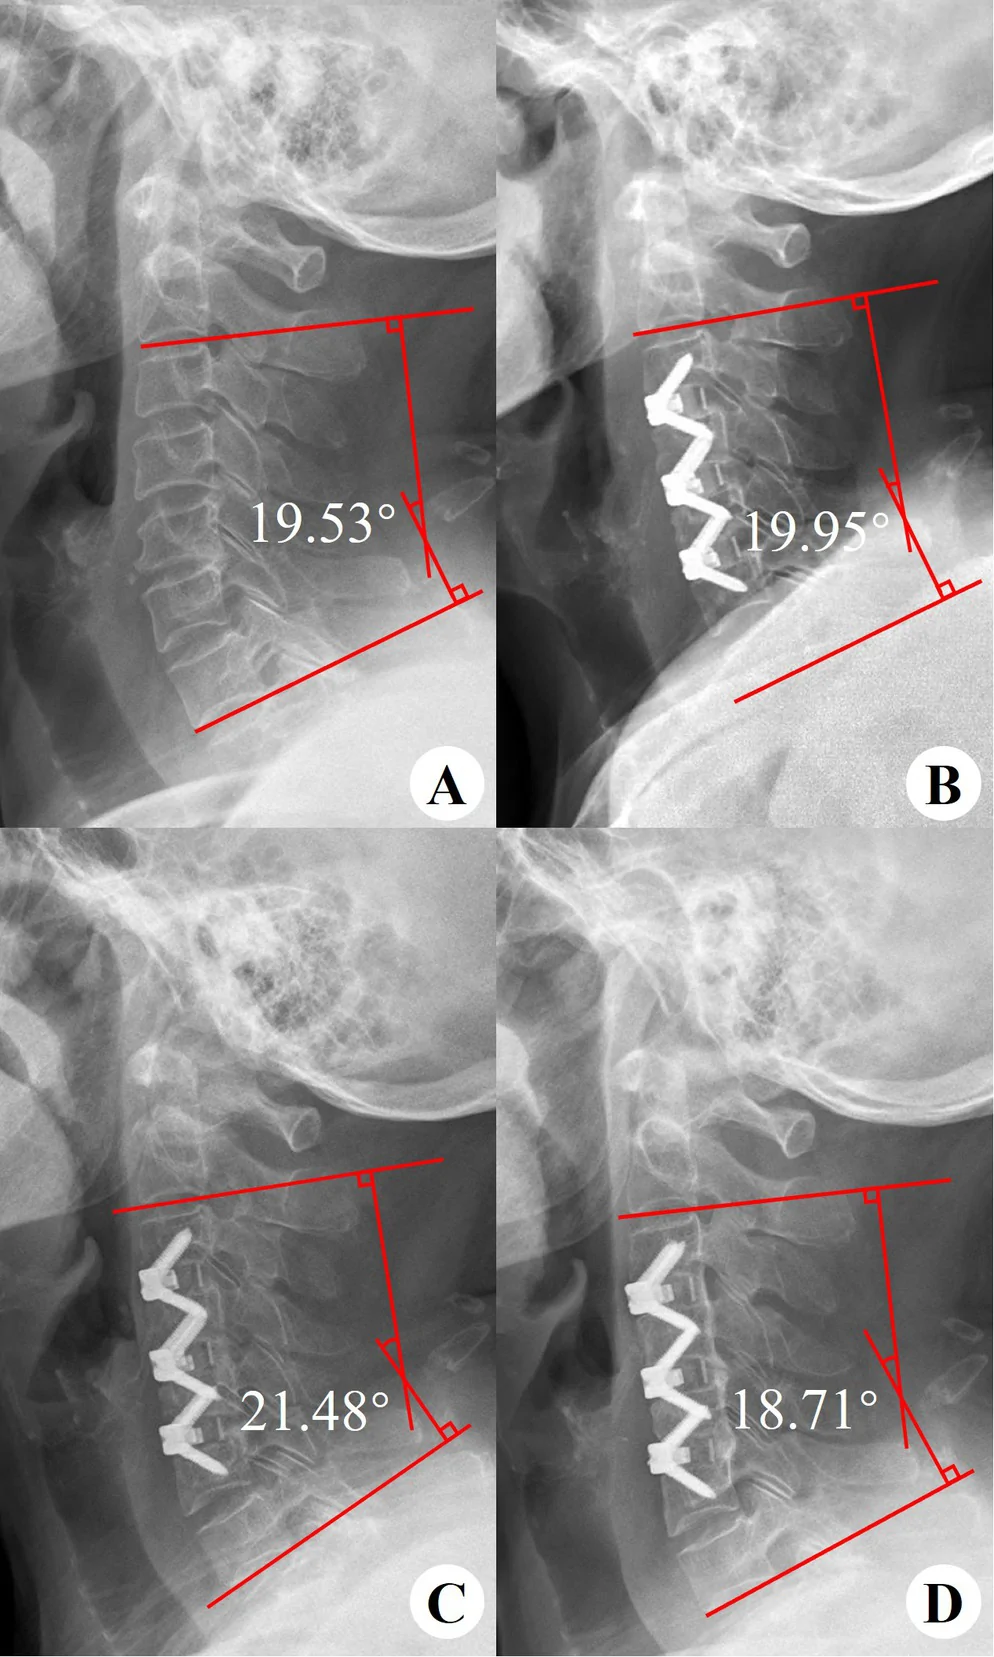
A 71‐year‐old woman with 3‐level CDDD, 2 years after ACDF with Zero‐p device. (A) Preoperative radiograph shows C2‐7 Cobb angle of 19.53°. (B) Radiograph at 1‐week post‐operation shows C2‐7 Cobb angle of 19.95°. (C) Radiograph at 3 months post‐operation shows C2‐7 Cobb angle of 21.48°. (D) Radiograph at 25 months post‐operation shows curvature loss of 1.24° compared to early post‐operation, but maintained at 18.71°.
